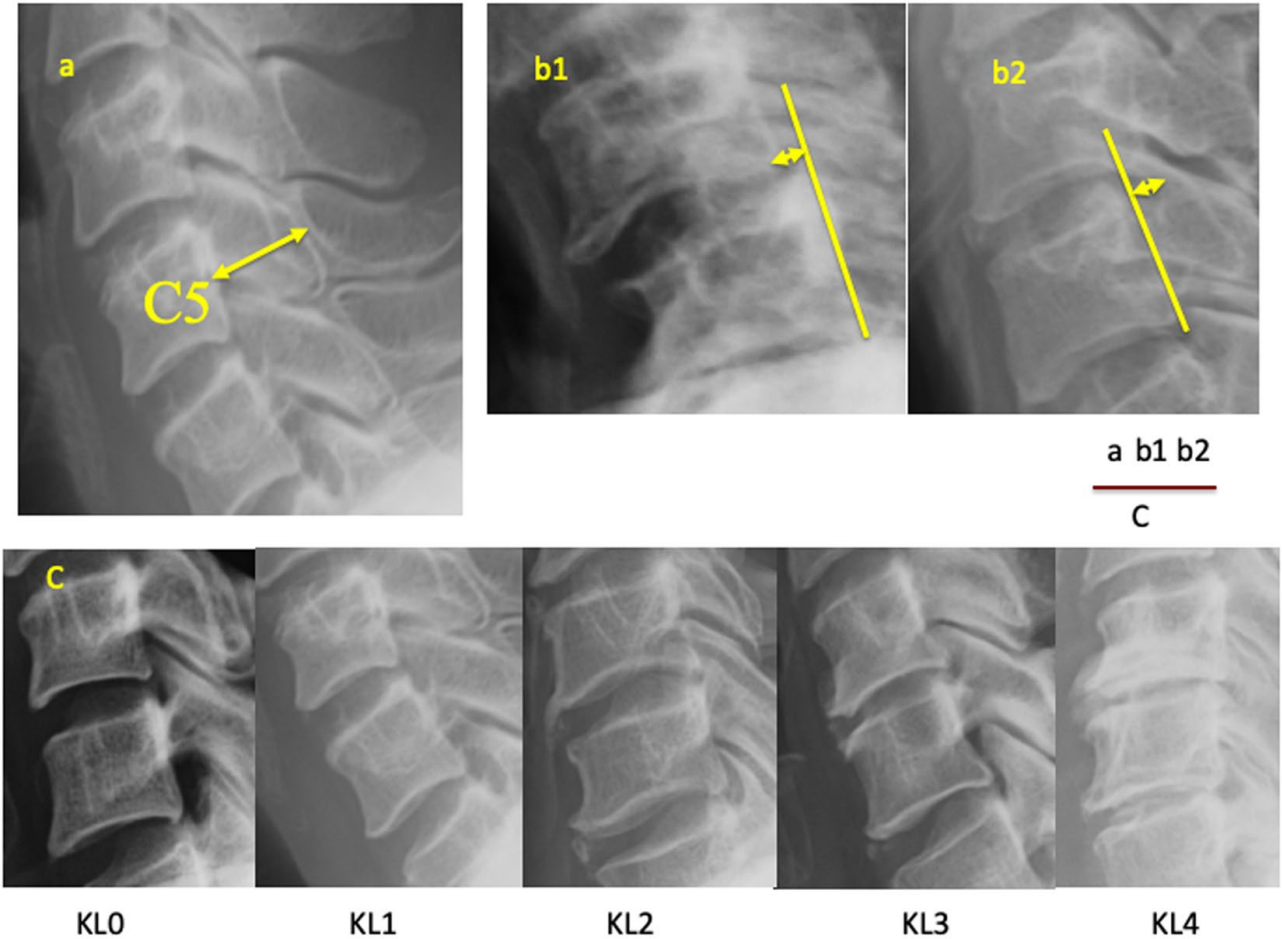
Radiographic measurements of the cervical spine. (a) Diameter of the cervical spinal canal at C5. (b) Measurement of cervical anterior/posterior spondylolisthesis. (c) Kellgren-Lawrence classification for grading of cervical spondylosis.
